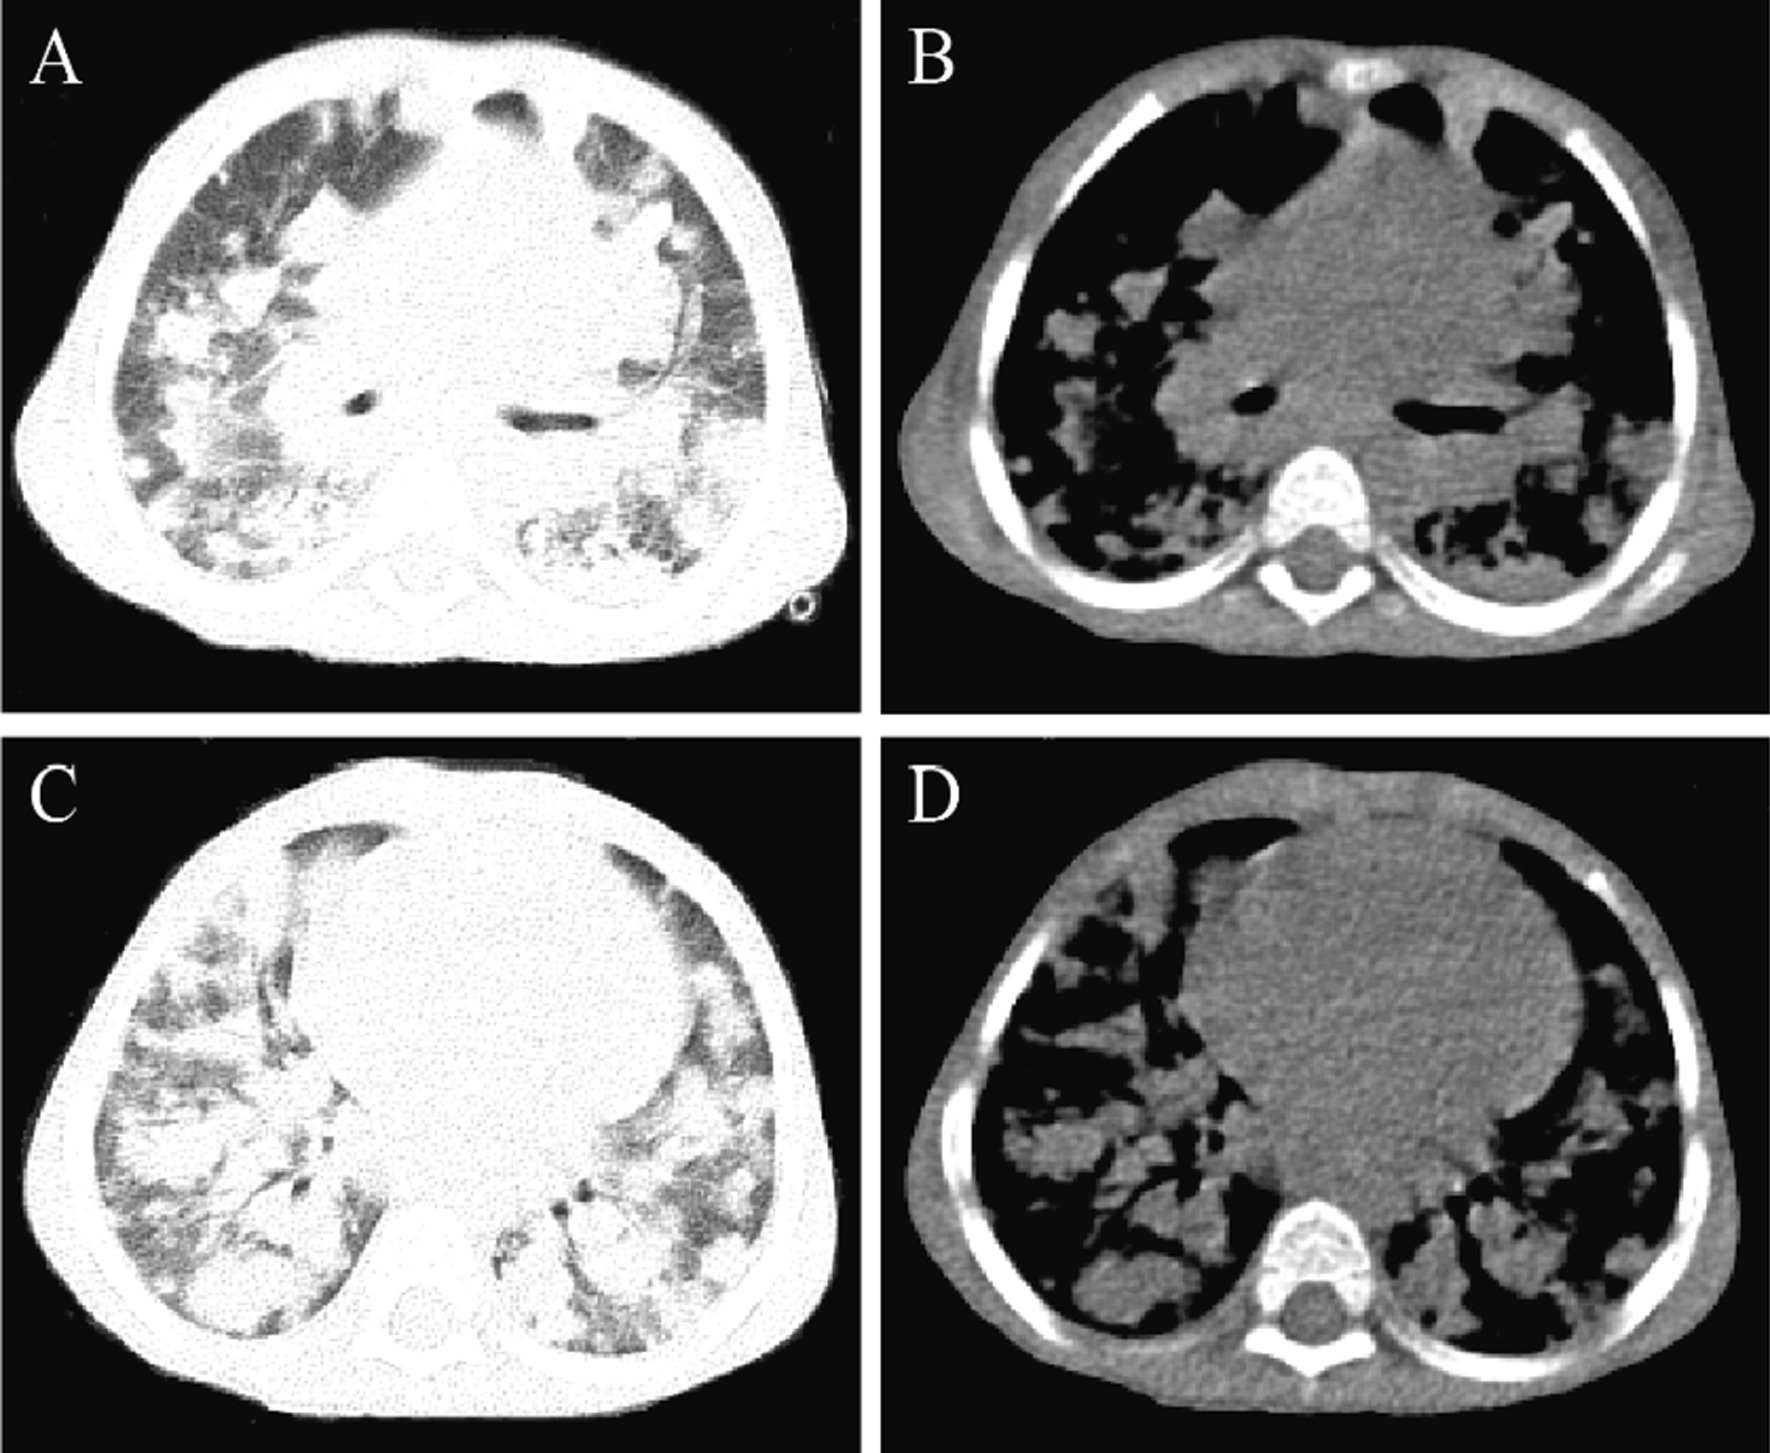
Chest CT showed multiple clusters and nodular high-density shadows in both lung fields. A and C were lung window images; B and D were corresponding mediastinum window images
